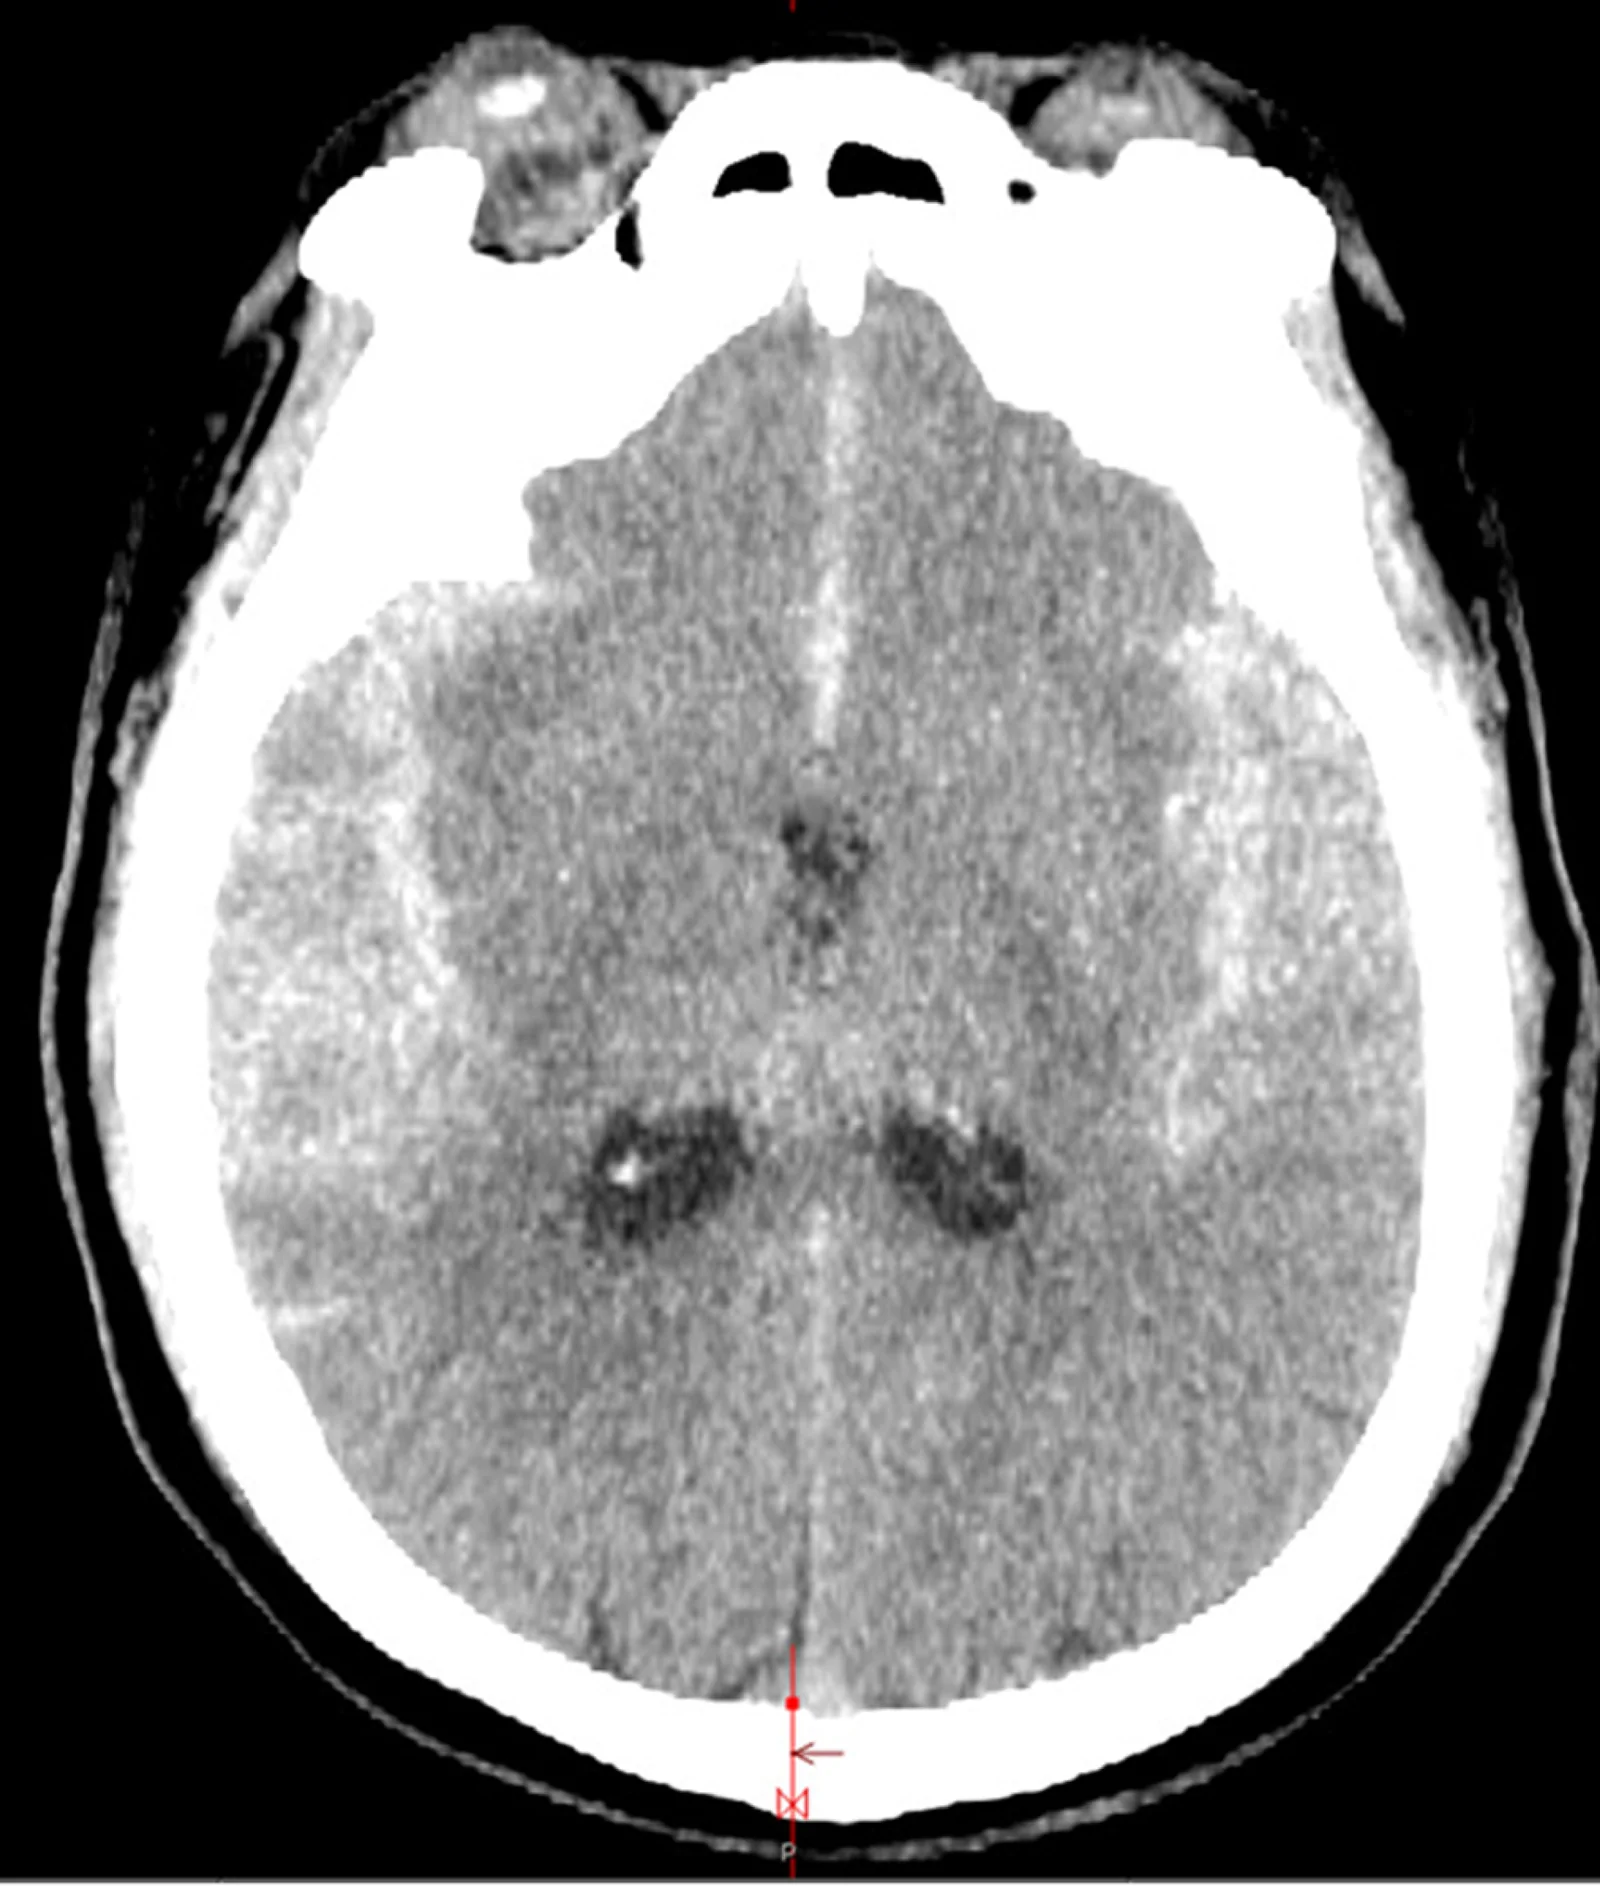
A CT scan of the head without contrast (axial) shows diffuse subarachnoid hemorrhage.
